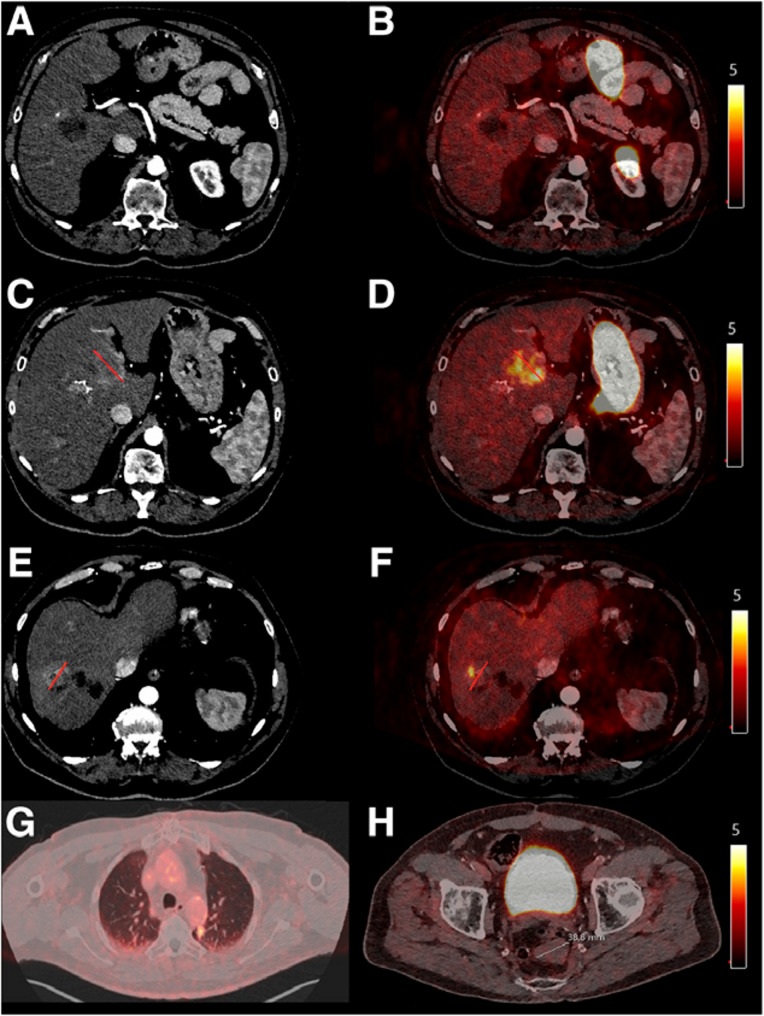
[^68^Ga]Ga-RAYZ-8009 PET/CT imaging in a 66-year-old patient with HCC. The images demonstrate high and specific tracer uptake in GPC-3-positive lesions, including intrahepatic tumors (C, D) and distant metastases (G). Conversely, no significant uptake is observed in biopsy-proven GPC-3-negative lesions, such as an HCC tumor in segment 5/6 (A, B) and a prostate cancer metastasis (H), confirming the tracer’s high specificity in vivo. Red arrows indicate the corresponding lesions and focal accumulations (Arrows have been enhanced for better visibility). (This research was originally published in JNM. Poot AJ, et al. [56] [^68^Ga]Ga-RAYZ-8009: A Glypican-3–Targeted Diagnostic Radiopharmaceutical for Hepatocellular Carcinoma Molecular Imaging—A First-in-Human Case Series. J Nucl Med. 2024;65:1597–1603. © SNMMI.)
